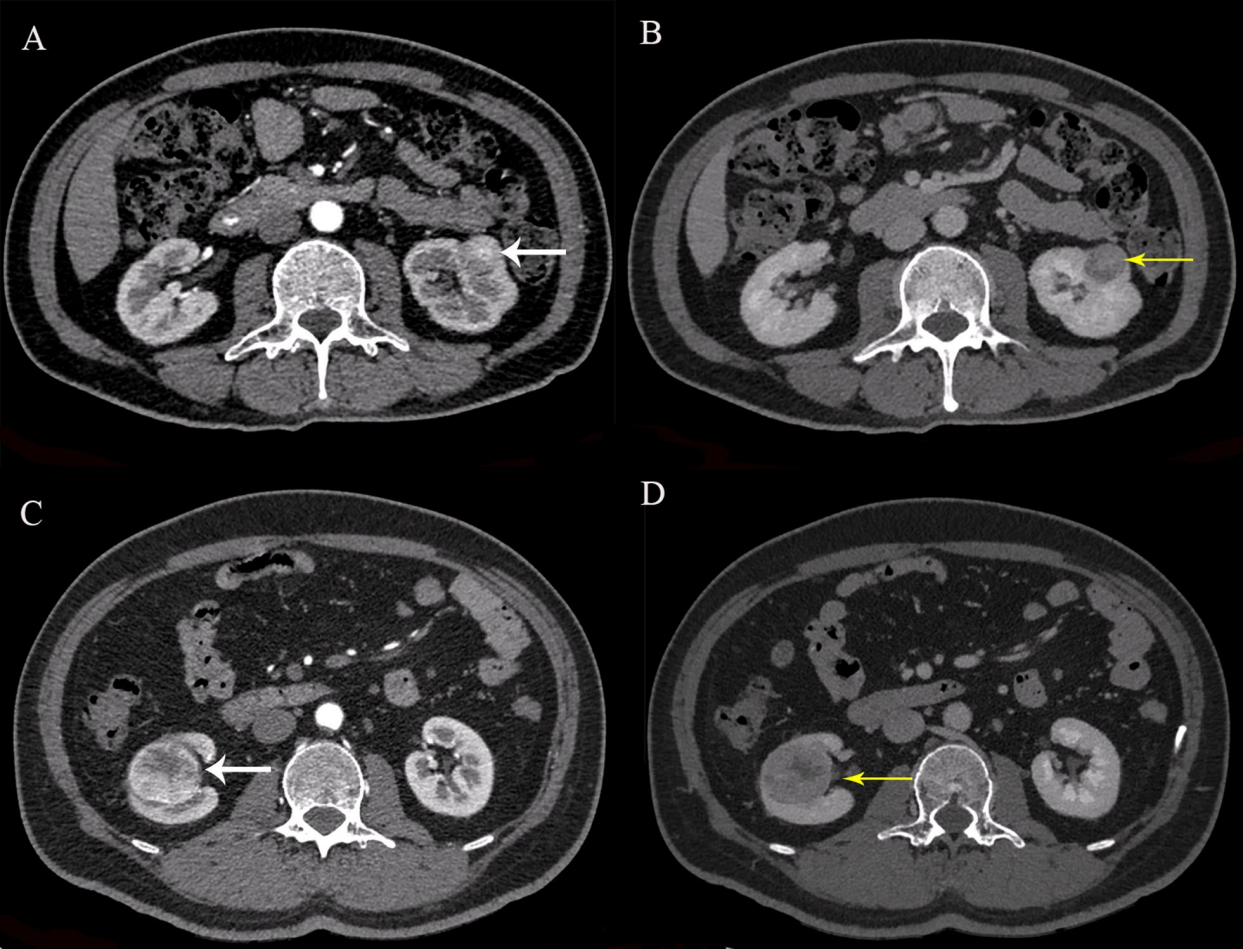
Images showed that a 58-year-old man had clear cell carcinoma of the left kidney with a diameter of 23.9 mm (A, B). The CT value of the lesion in the corticomedullary phase image (A) was similar to that of normal renal cortical enhancement. The lesion was not significant in the corticomedullary phase but could be clearly displayed on 60-keV nephrographic phase VMIs (virtual mono-energetic images) (B). Images showed that a 47-year-old man had clear cell carcinoma of the right kidney with a size of 41.4 mm × 35.0 mm (C, D). The lesion visibility was superior on corticomedullary phase images (C) than on 60 keV VMIs (D)
